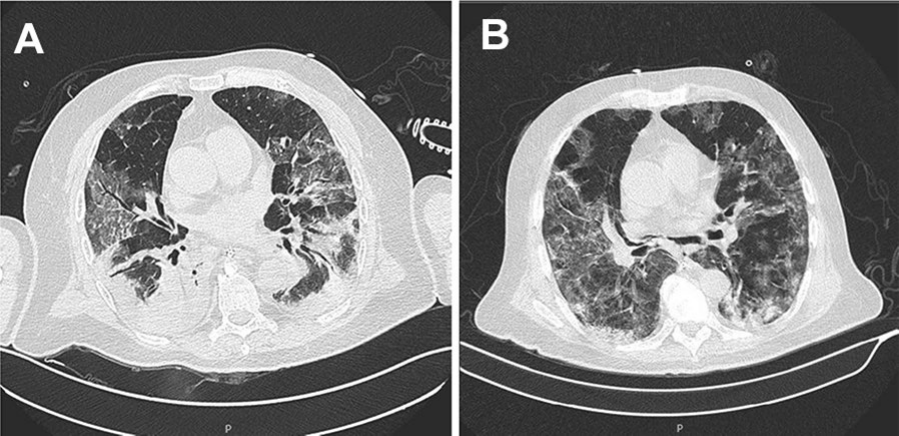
A CT on the admission day. CT showing ground glass opacity (GGO) areas with the presence of consolidation areas in the posterior region. B CT after 22 days of hospitalization. CT with replacement of the consolidation for ground glass opacity (GGO) areas, demonstrating significant improvement of the clinical condition
